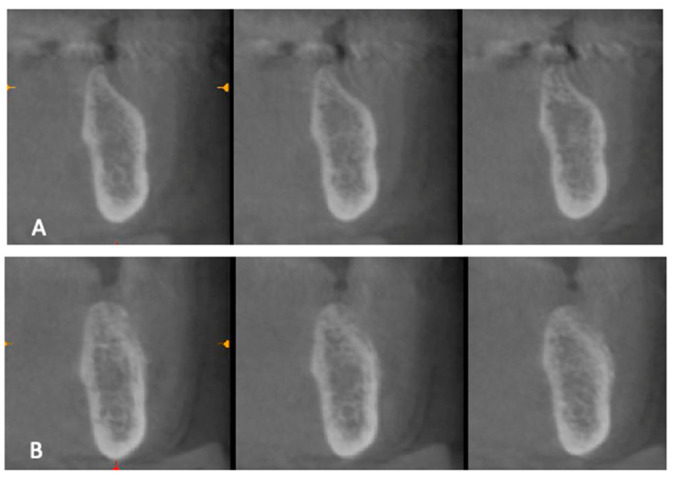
Radiographic investigation before surgery (A) and after the healing time (B) before implants.
